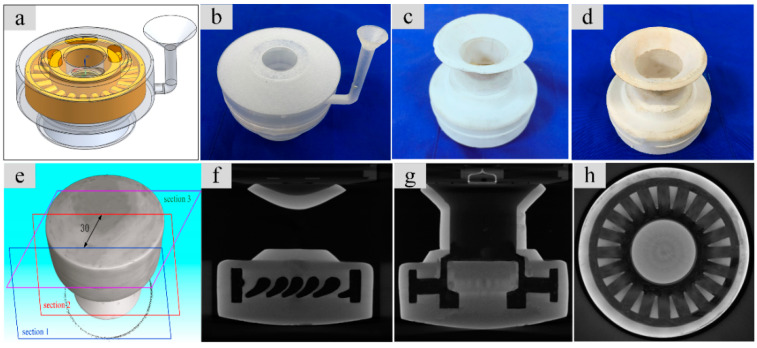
Fabrication of CaO-based ceramic mold of an impeller and the CT image of mold: (a) CAD mold, (b) resin mold, (c) dried green body, (d) sintered ceramic mold, (e) scan section of CT, (f) CT image of Section 1, (g) CT image of Section 2, (h) CT image of Section 3.
